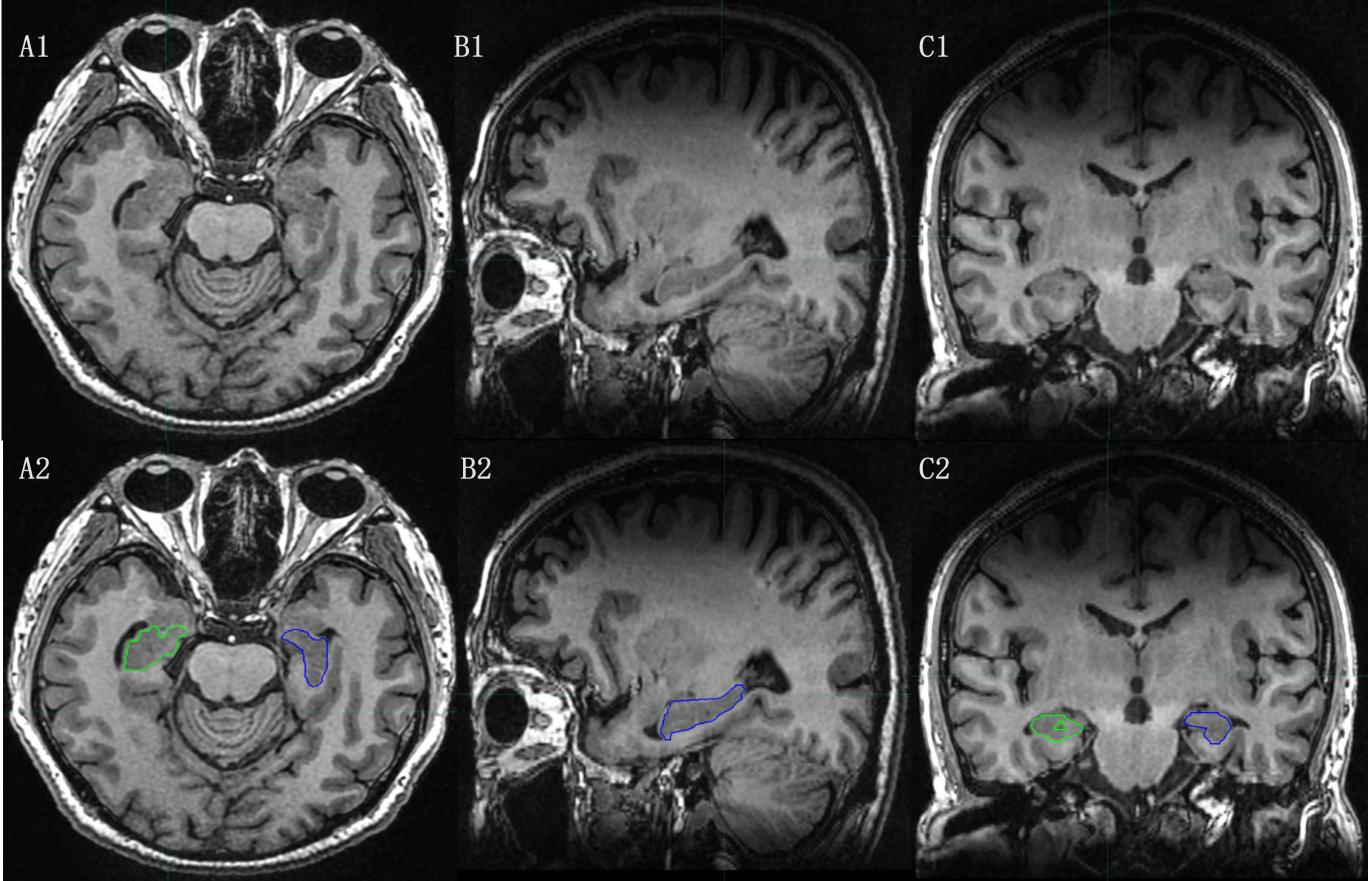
The hippocampal contour based on MRI T1-weighted Images. [(A1), (B1), (C1):Axial, Sagital and Coronal images of MRI T1-weighted Images; (A2), (B2), (C2): Axial, Sagital and Coronal images of MRI T1-weighted Images with hippocampal contour).
